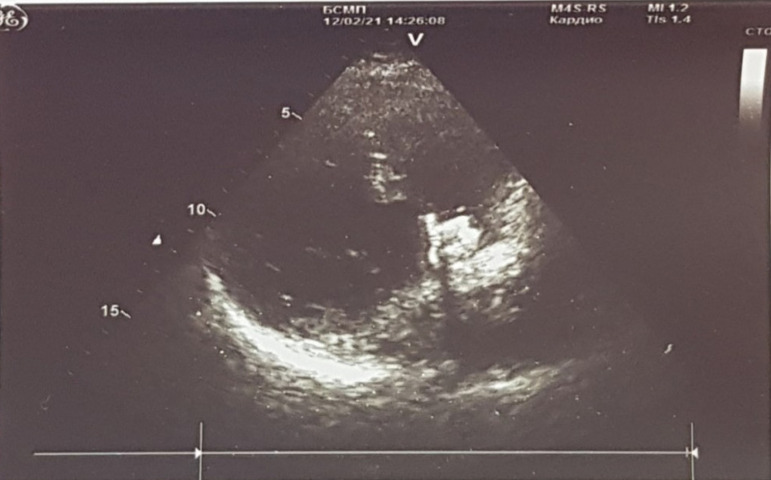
Echocardiography one month after surgery.
